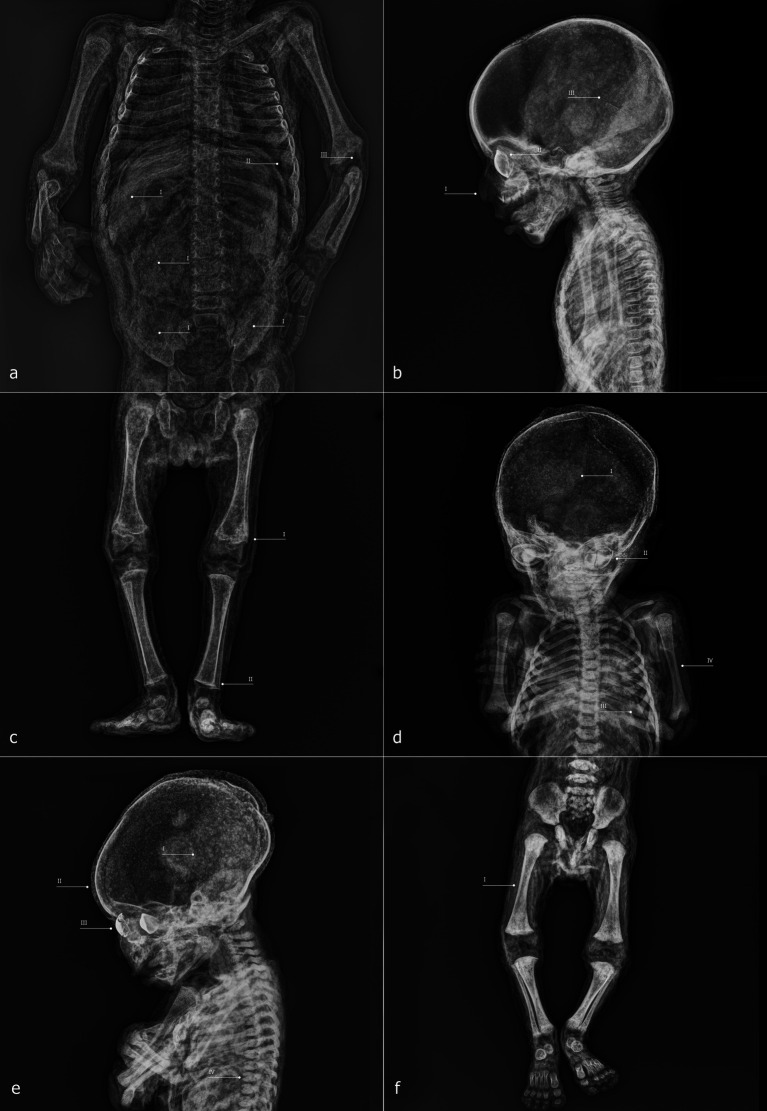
ID3—radiographic images: anteroposterior view of the trunk and upper limbs (a); laterolateral view of the skull and torso (b); anteroposterior view of the lower limbs (c). ID4—radiographic images: anteroposterior view of the skull and trunk (d); laterolateral view of the skull and torso (e); anteroposterior view of the lower limbs (f).
